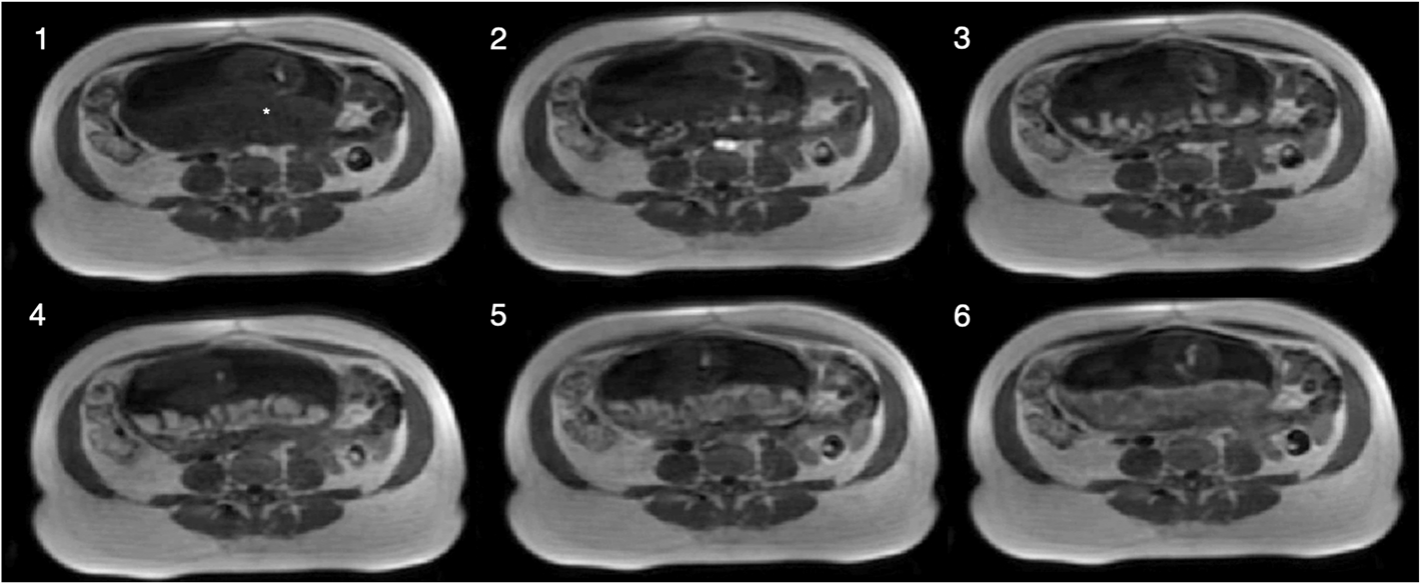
Dynamic enhancement of the placenta with DCE‐MRI. Baseline image (1), arrival and wash‐in (2‐4), wash‐out (5‐6)
